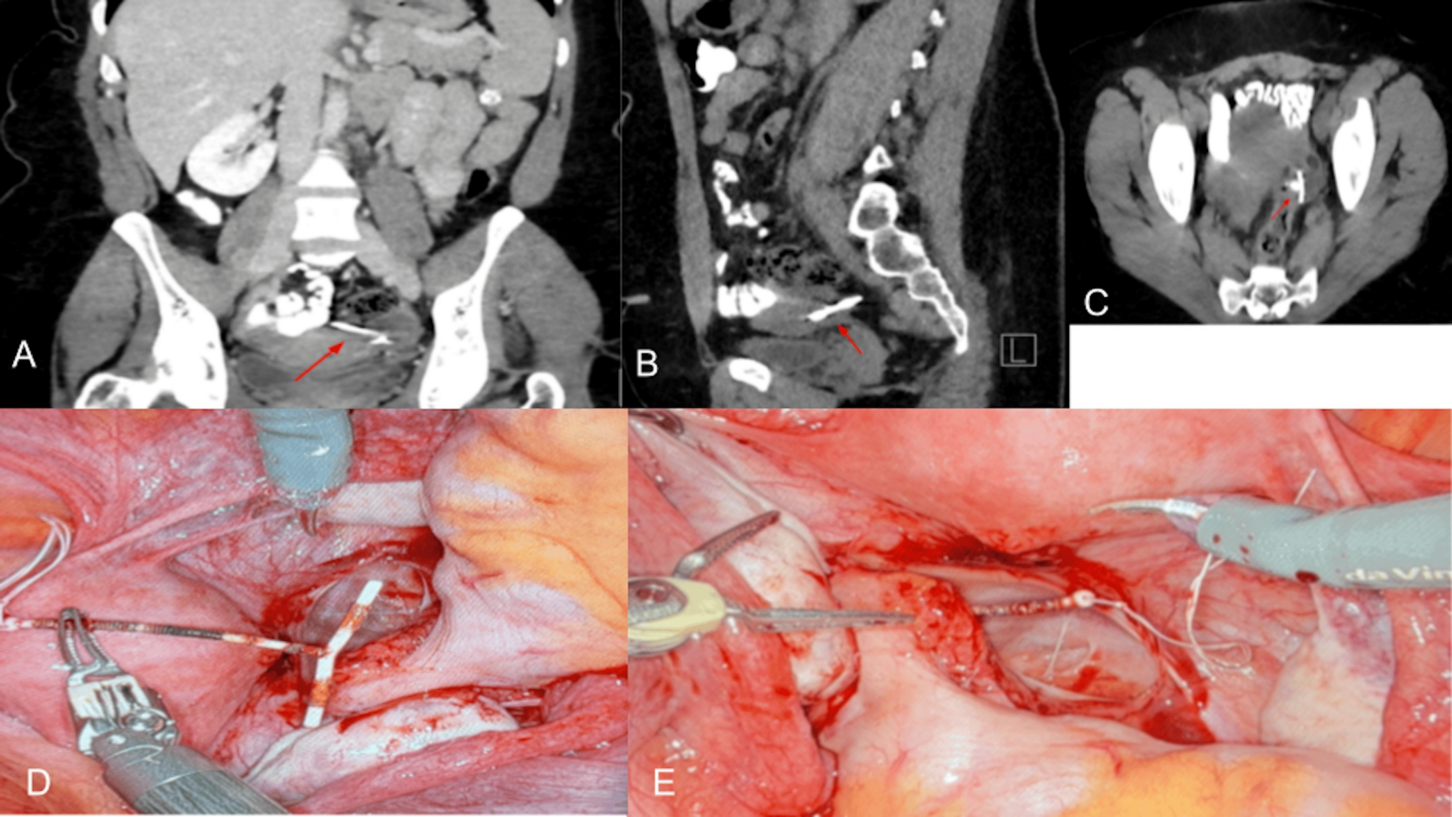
(A) Coronal (frontal plane), (B) sagittal, and (C) axial CT scan showing misplaced intrauterine device (IUD) within the pelvis, positioned posterior to the uterus and adjacent to the rectum. (D, E) Intraoperative view demonstrating the extraction of an intrauterine device (IUD) during robotic-assisted pelvic surgery.
